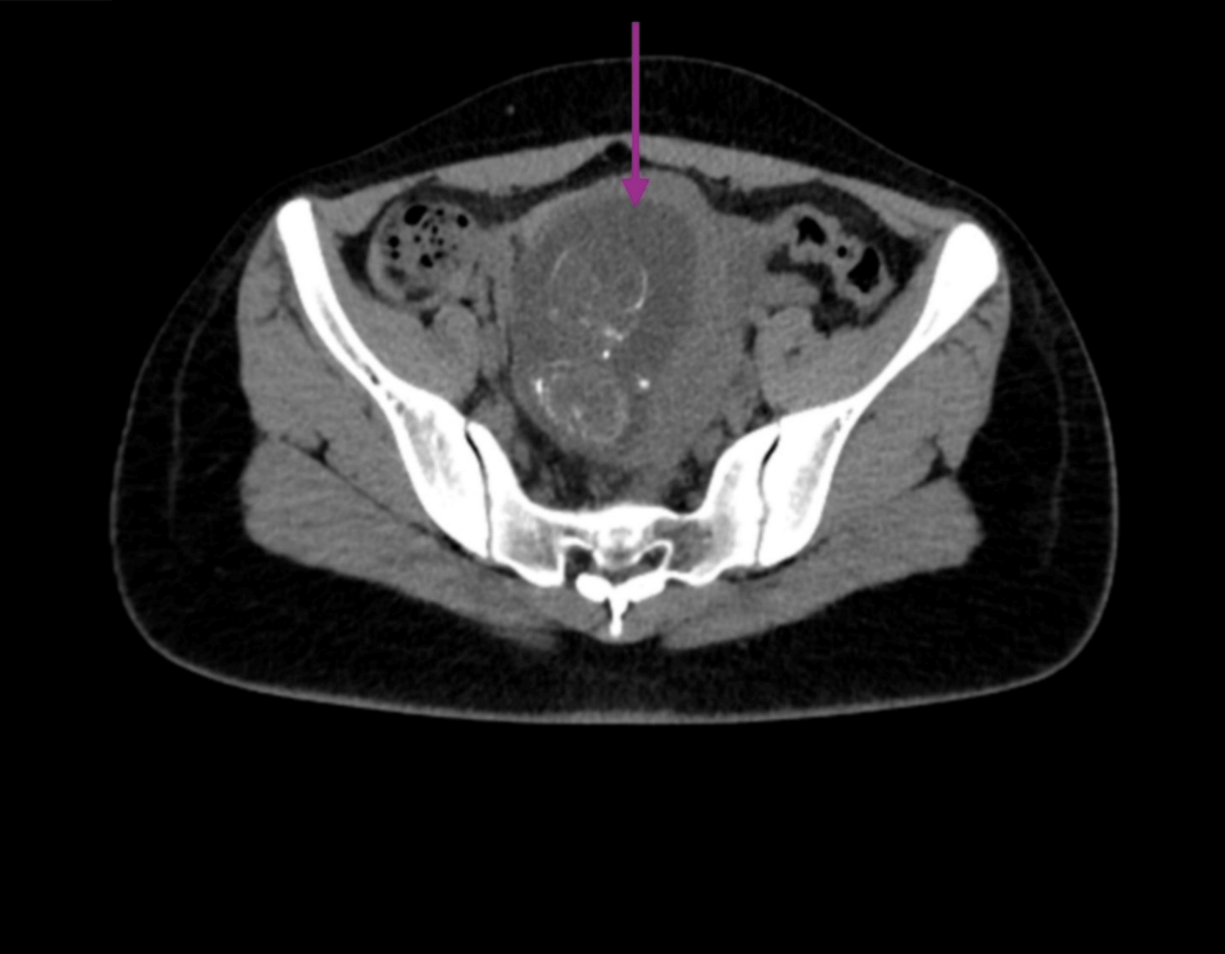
Computed tomography scan confirming extrauterine pregnancy (purple arrow)
